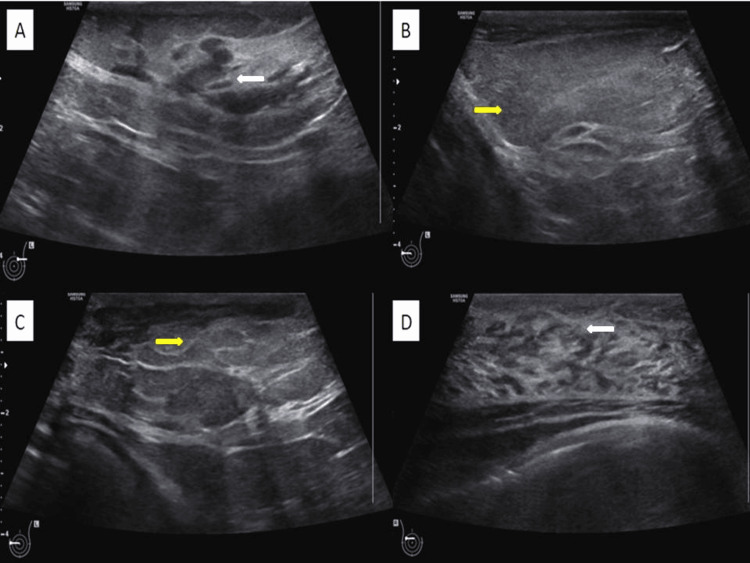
A) High-resolution ultrasound of the left breast upper outer quadrant showing an asymmetrical distribution of fibro-glandular tissue (white arrow). B) and C) The rest of the left breast was showing fatty breast tissue (yellow arrow). D) Normal fibro-glandular tissue seen in the contralateral breast (white arrow).
